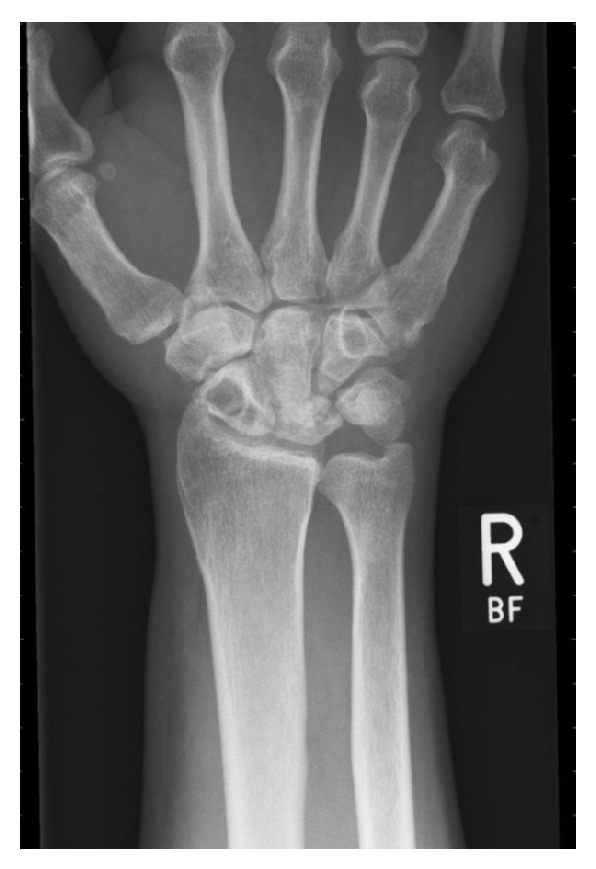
X-Ray: cystic scaphoid changes and partial lunate collapse.
